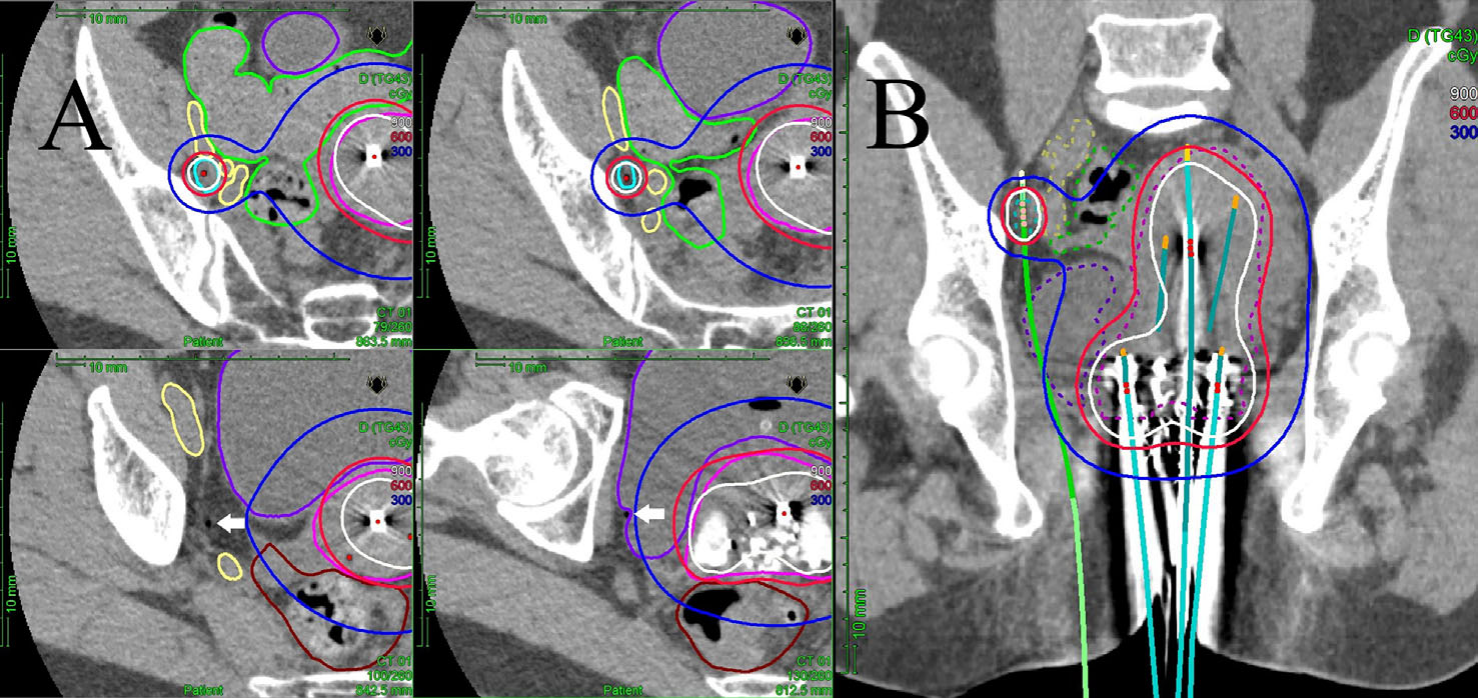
(A) Multi-slice axial CT images of the right obturator node (cyan line), uterine target (pink line), bowel (green line), external/internal iliac vessel (yellow line), rectum (brown line), and bladder (purple line) receiving 50% (blue lines), 100% (red lines), and 150% (white lines) of the prescription dose (6 Gy) in a representative patient with a right obturator node metastasis. One interstitial flexible catheter (white arrows) was inserted in the lithotomy position passing from the inside of the pubic bone through the outside of the bladder up to the front of the right sacroiliac joint using TRUS and CT guidance in the HDR room with dedicated CT, while avoiding bladder and external/internal iliac vessels. TRUS is commonly used for implantation from the perineum, and CT is used to adjust catheter positions at a deeper level. An iterative approach of catheter adjustment and CT image acquisition allows precise placement of the catheter in the target volume. (B) A coronal CT image of the uterine target (pink dashed line), the right obturator node (cyan dashed line), bowel (green dashed line), external/internal iliac vessel (yellow dashed line), rectum (brown line), and bladder (purple dashed line) receiving 50% (blue lines), 100% (red lines), and 150% (white lines) of the prescription dose (6 Gy) from interstitial and intracavitary applicators in a representative patient with a right obturator node metastasis. It is apparent that one interstitial flexible catheter (light green) is implanted, passing from the inside of the pubic bone through the outside of the bladder, so that the catheter can be inserted to the central part of the node.
